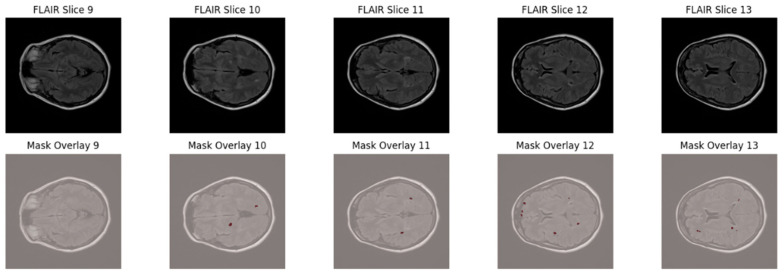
Sequential FLAIR slices from a single MS patient (slices 9–13) with manually annotated lesion overlays shown in red.
